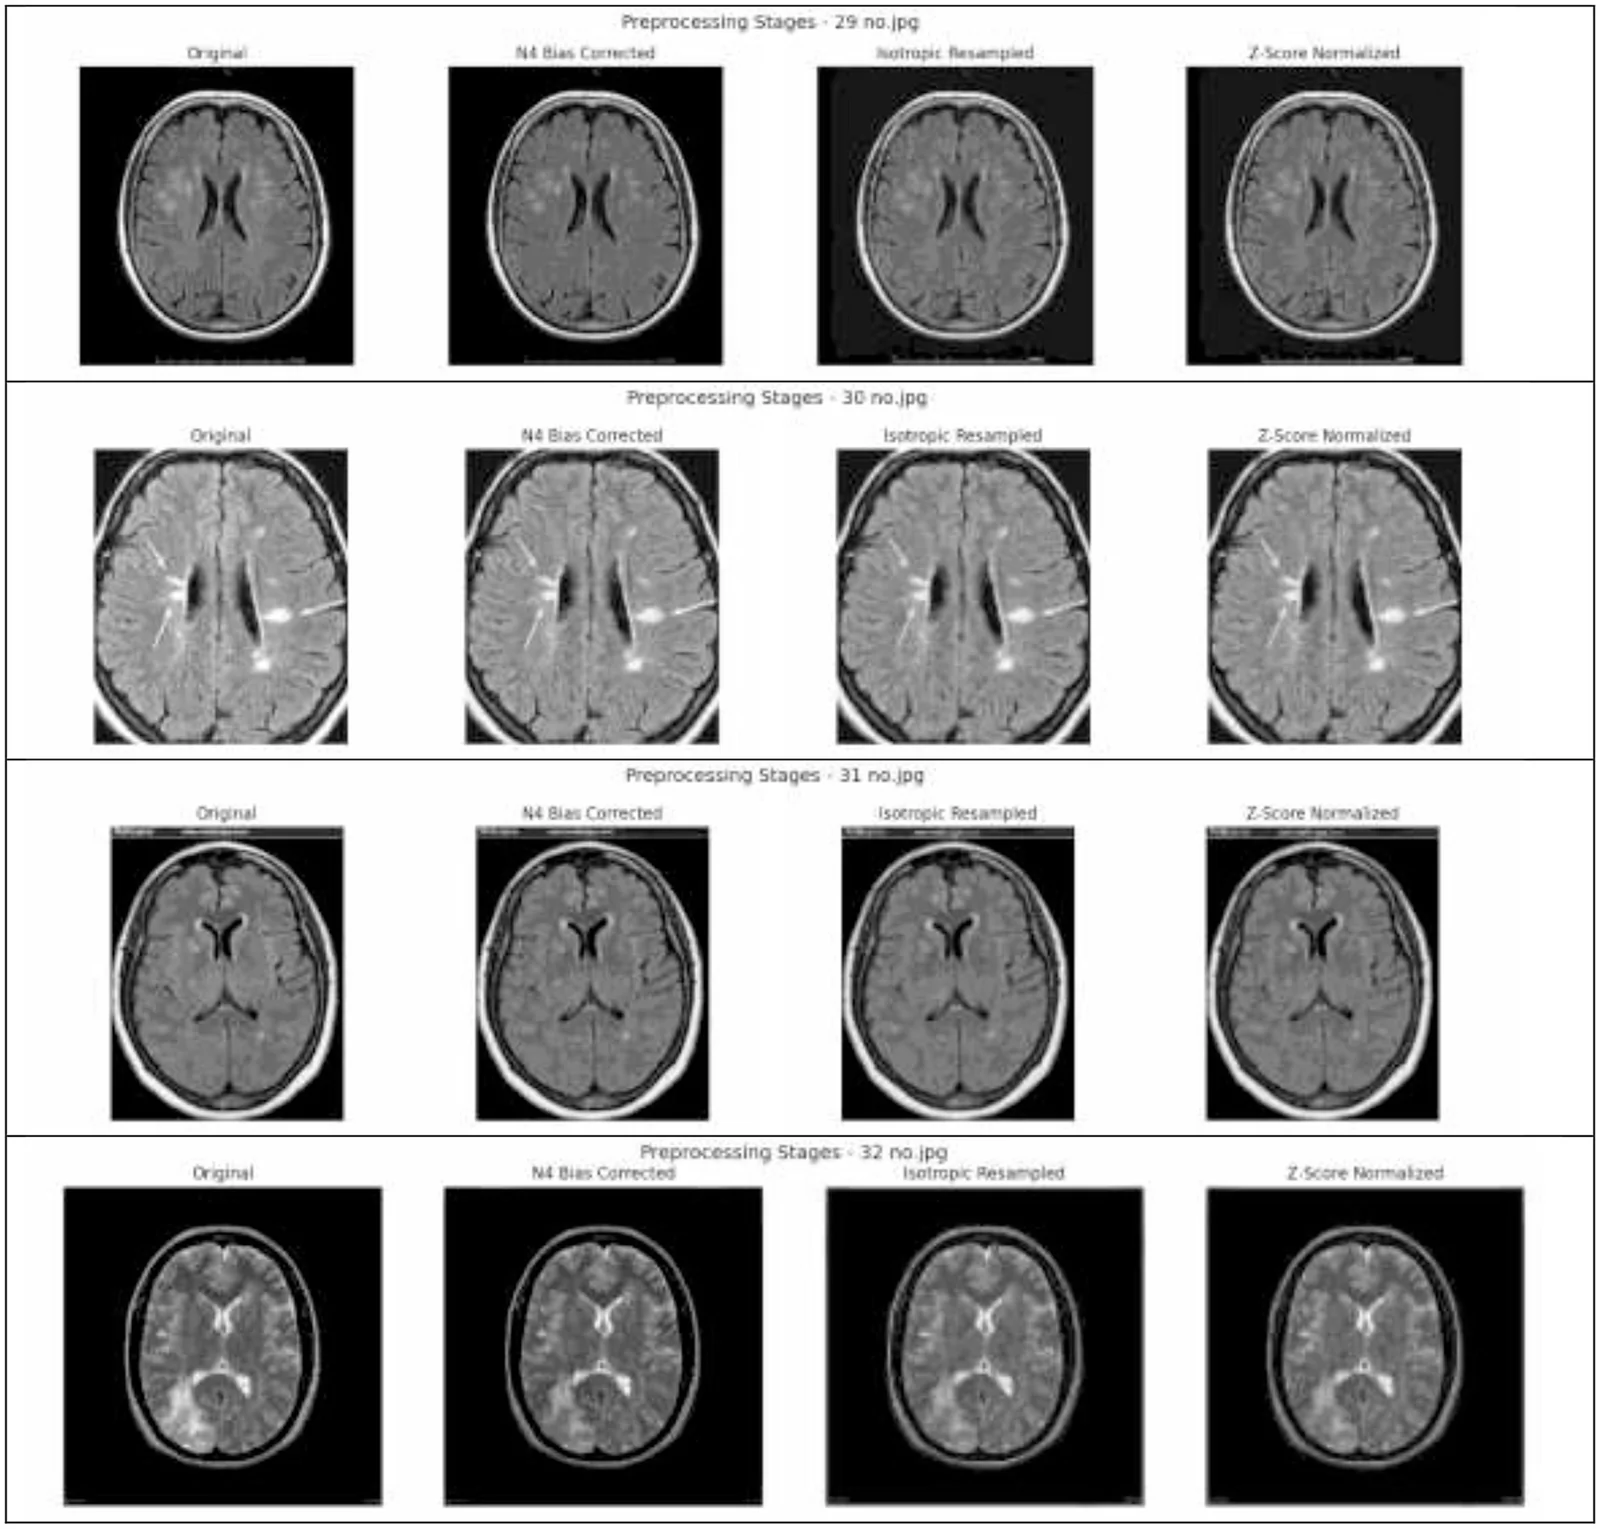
Preprocessed images of the CT dataset.
